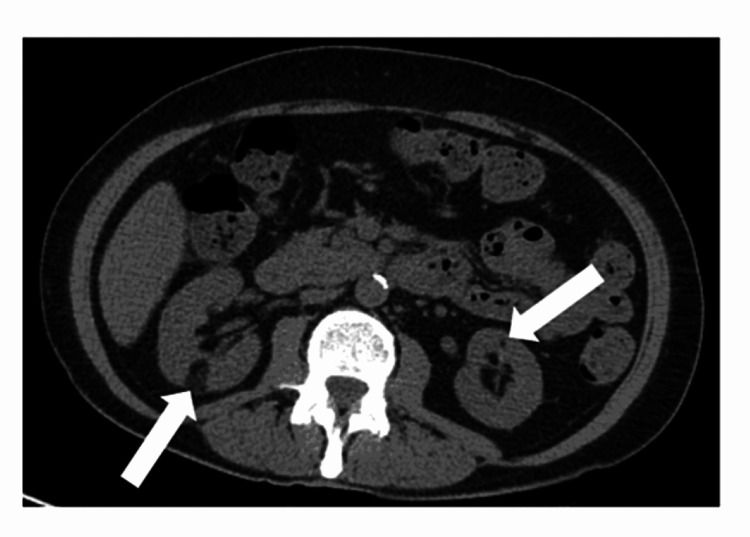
Non-contrast CT image of a woman with polycythemia rubra vera reveals bilateral fat-containing renal neoplasms interpreted as sporadic angiomyolipomas (arrows). The diameter of the largest neoplasm was 1.6 cm (right kidney).
